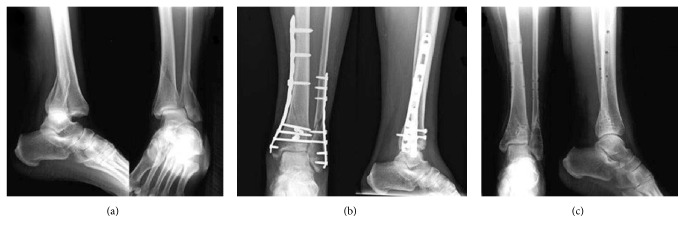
Case N*°* (42) of Table 2:* a 41-year-old woman with a 43-B1 closed fracture treated with* MIPO technique:* (a) preoperative radiographic image; (b) postoperative radiographic image at 1-month follow-up; (c) radiographic aspect at 39-month follow-up after implant removal.
